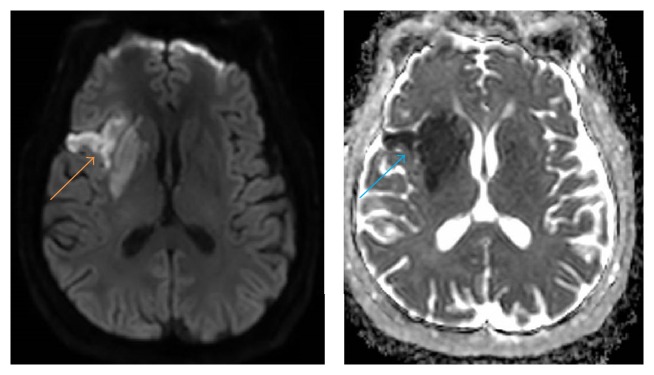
MRI brain reveals an area of diffusion restriction over the right MCA territory (orange arrow) with corresponding signal loss on Apparent Diffusion Coefficient (ADC) mapping (blue arrow), suggesting acute ischemic infarct.
